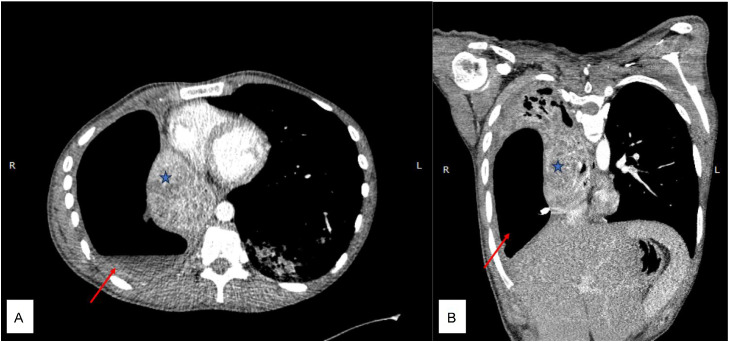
Chest computed tomography with contrast mediastinum window with axial (A) and coronal view (B), indicating large right pyopneumothorax (red arrow), right lobe collapse (blue star) and right lobe cavity continuing into the pleural area, suggesting a bronchopleural fistula.
